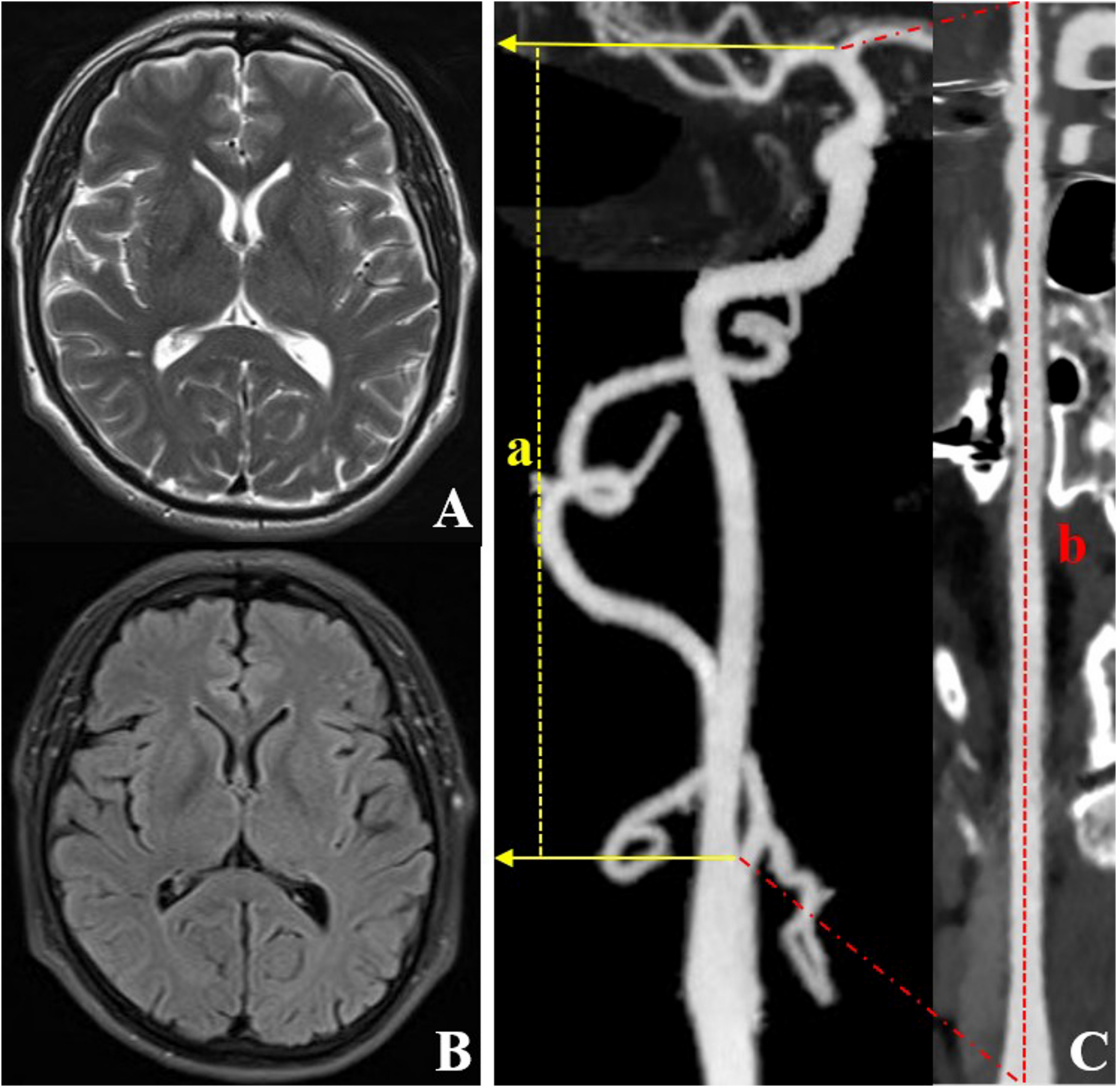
CTA and MRI images of an individual (a 59-year-old male) with right ICA TI equal to 1.33. T2WI (A) and FLAIR (B) show a grade 0 EPVS in the basal ganglia region. CTA with MIP (C) shows the lower tortuosity of the right ICA. The dotted line a represents the straight length of the ICA, and the dotted line b represents the centerline vessel length of ICA reconstructed by using a curved planar reformat. CTA, computed tomography angiography; EPVS, enlarged perivascular spaces; ICA, internal carotid artery; MIP, maximum intensity projection; MRI, magnetic resonance imaging; TI, tortuosity index.
